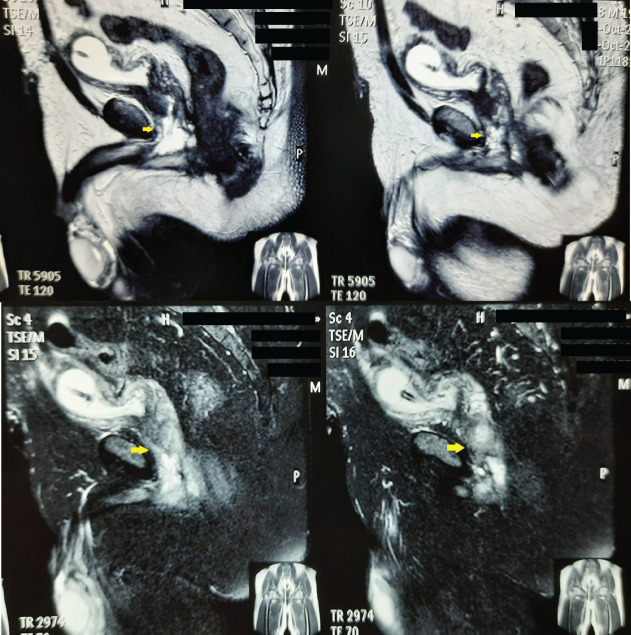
MRI images depicting a T2 hyperintense infiltrative mass involving the membranous urethra.
